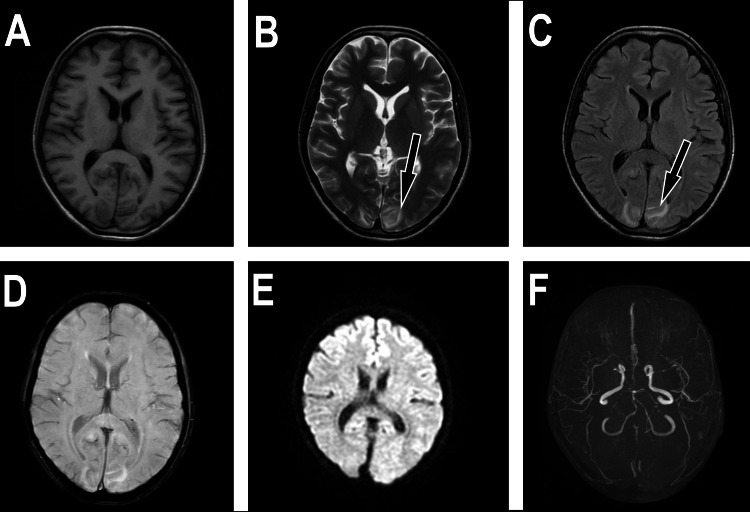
Axial MRI images of brain without contrast. The study demonstrated cortical and subcortical abnormal hypointensity on the T1-weighted image (A), hyperintense signal intensity on the T2-weighted image (B), and fluid-attenuated inversion recovery (FLAIR) (C) (arrows on B and C). There is no evidence of intraparenchymal hemorrhage on the susceptibility-weighted image (SWI) (D) or restricted diffusion on diffusion-weighted imaging (E). Time of flight MR angiography (TOF MRA) showed vascular irregularity. All images are the original work of the author.
